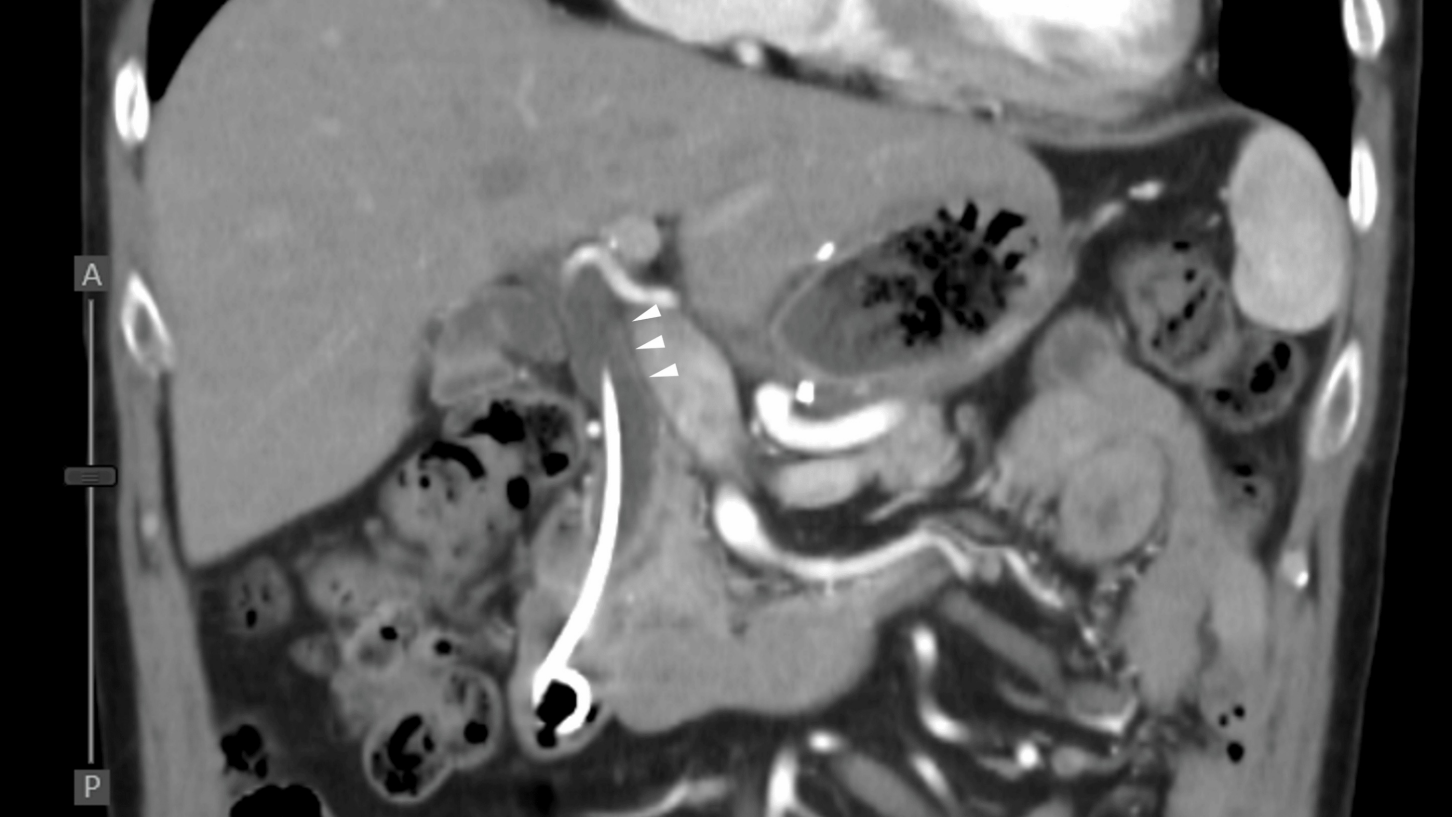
Coronal imaging of abdominal contrast-enhanced computed tomography eight months after immune-related sclerosing cholangitis Coronal imaging shows that although the common bile duct (CBD) dilation remained, diffuse CBD wall thickening improved (arrowheads).
